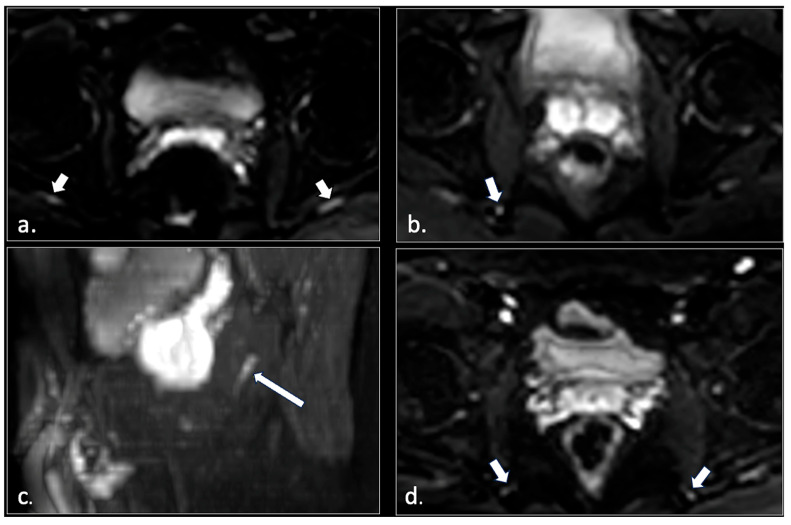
In Figure 10 are shown images related to diffusion-weighted imaging (DWI) scans. (a) does not show abnormalities of pudendal nerve. Note the physiological hyperintensity of the sciatic nerve (arrows). (b) depicts hyperintensity of the right pudendal nerve at the level of ischial spine. In the same patient, a sagittal reconstruction (long arrow in c) shows hyperintensity of the right pudendal nerve along the proximal Alcock’s canal. In (d) a case of bilateral hyperintensity of the nerve can be seen.
